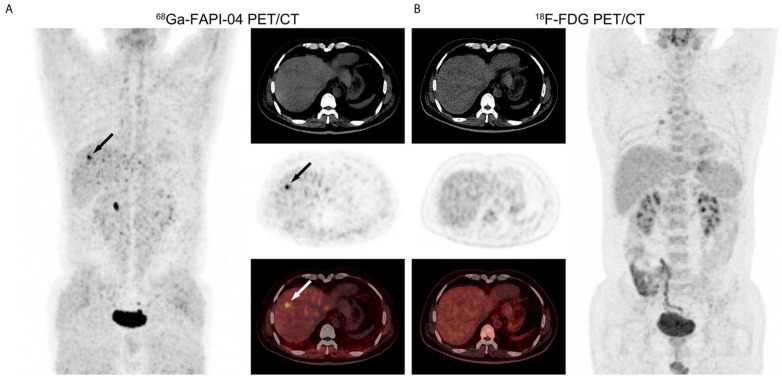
PET/CT scans of a 53-year-old male diagnosed with moderately differentiated HCC. (A) The [^68^Ga]Ga-FAPI-04 PET/CT detected a high-affinity lesion (black and white arrows, SUVmax = 7.36) in the liver’s right lobe. (B) In contrast, no abnormalities were identified in the liver on the [^18^F] FDG PET/CT images (SUVmax = 2.36). Images originally published by Wang et al. [152].
